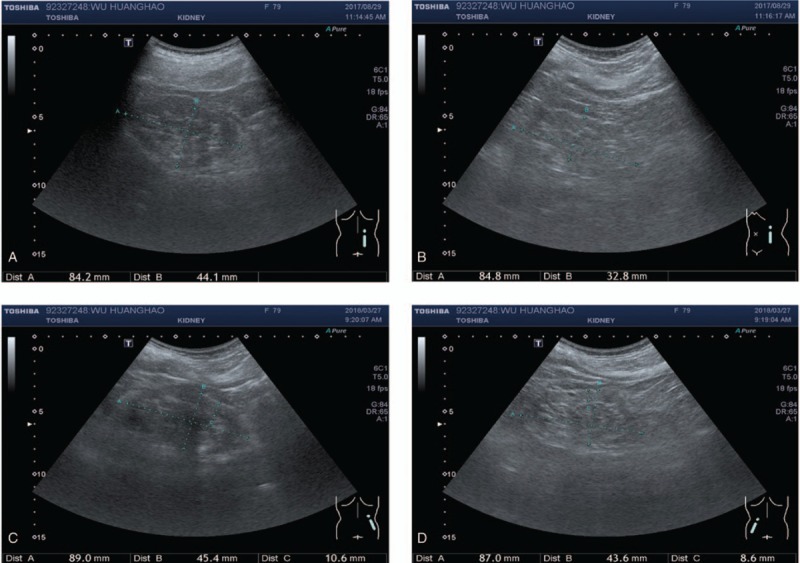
Ultrasonography scanning at outpatient clinic before and after Eefooton treatment. The patient had received the ultrasound scanning of both sides of kidney at outpatient clinic before the Eefooton treatment on August 29th 2017 (right: A, left: B) and at the end of 6-month Eefooton treatment on March 27th 2018 (right: C, left: D).
